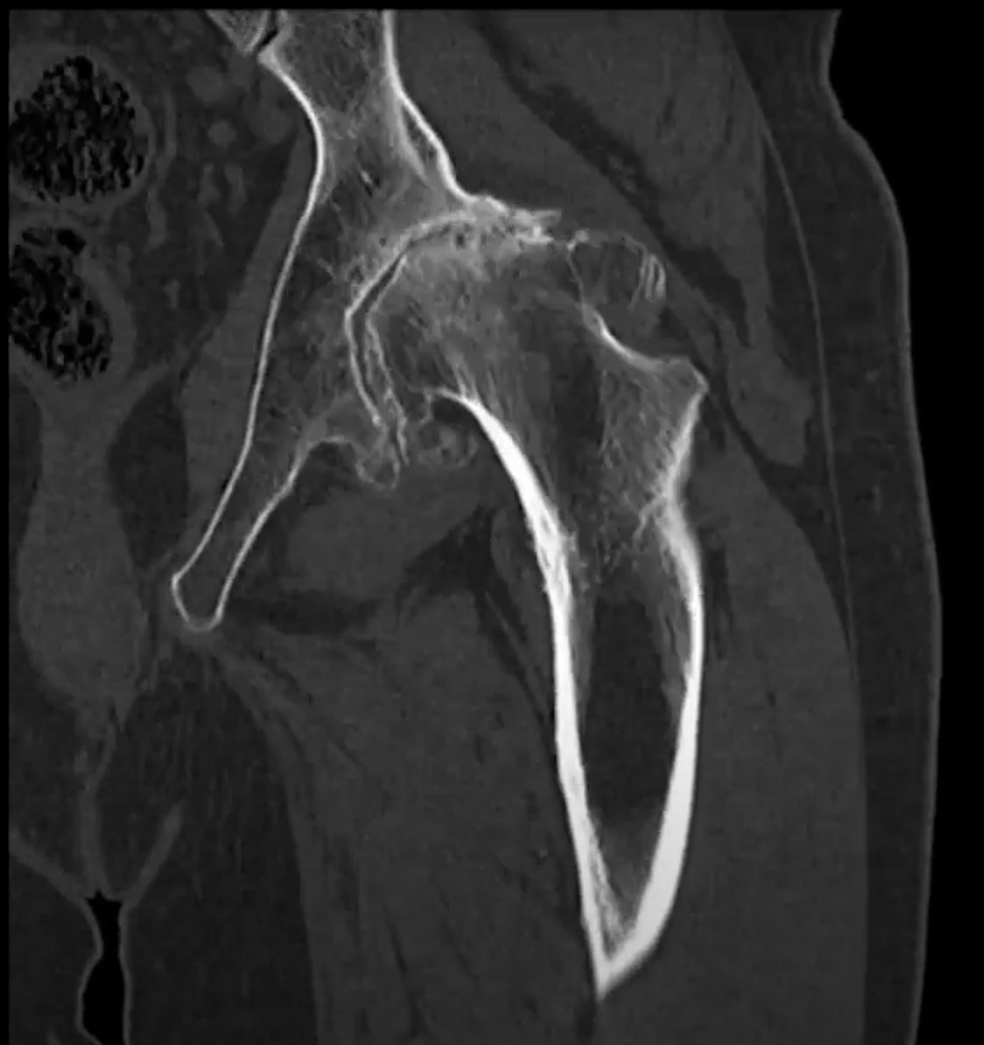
Coronal CT of the left hip and femoral acetabular joint.
